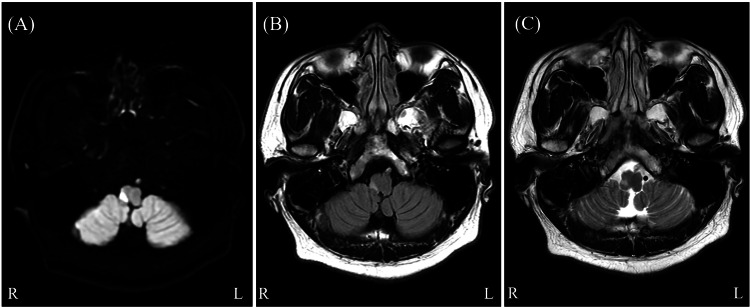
Brain magnetic resonance imaging (MRI) results. The brain MRI reveals hyperintensity in the right lateral medulla oblongata. (A) Diffusion-weighted imaging. (B) T2 fluid-attenuation inversion recovery imaging. (C) T2-weighted imaging.
